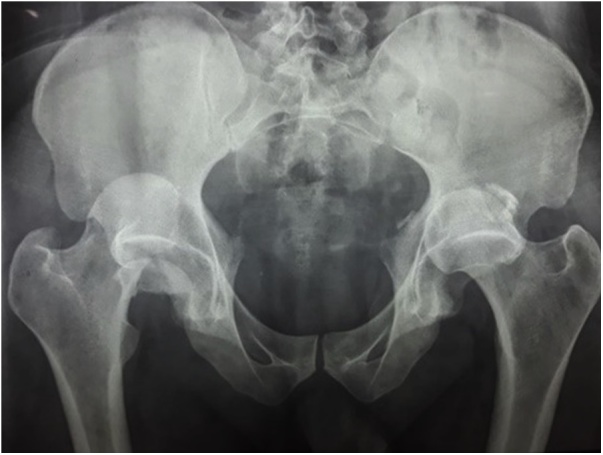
Anteroposterior radiograph of the pelvis showing bilateral posterior hip dislocation with right Pipkin type II fracture.
